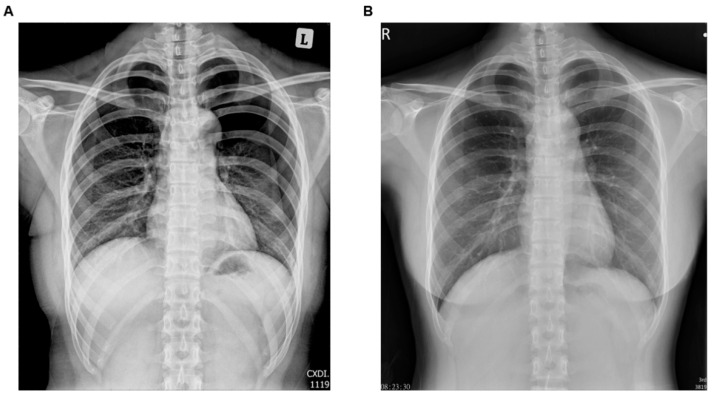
Chest X-ray (CXR) (A) after acupuncture treatment, the next day, and (B) when the patient was discharged from the hospital.
